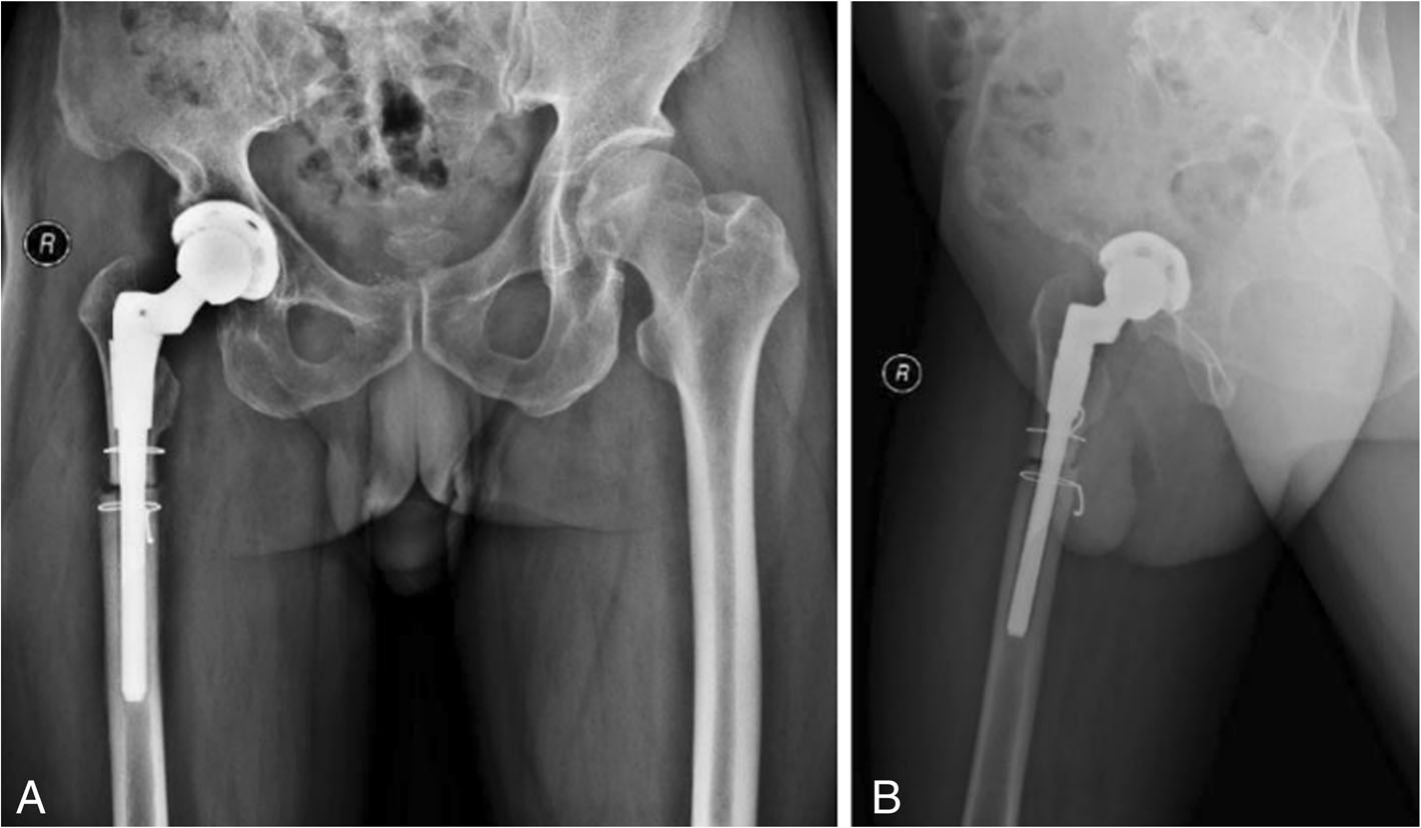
Radiographs of the right hip at 5 months after the operation. (a) anteroposterior view of the right hip, (b) lateral view of the right hip
